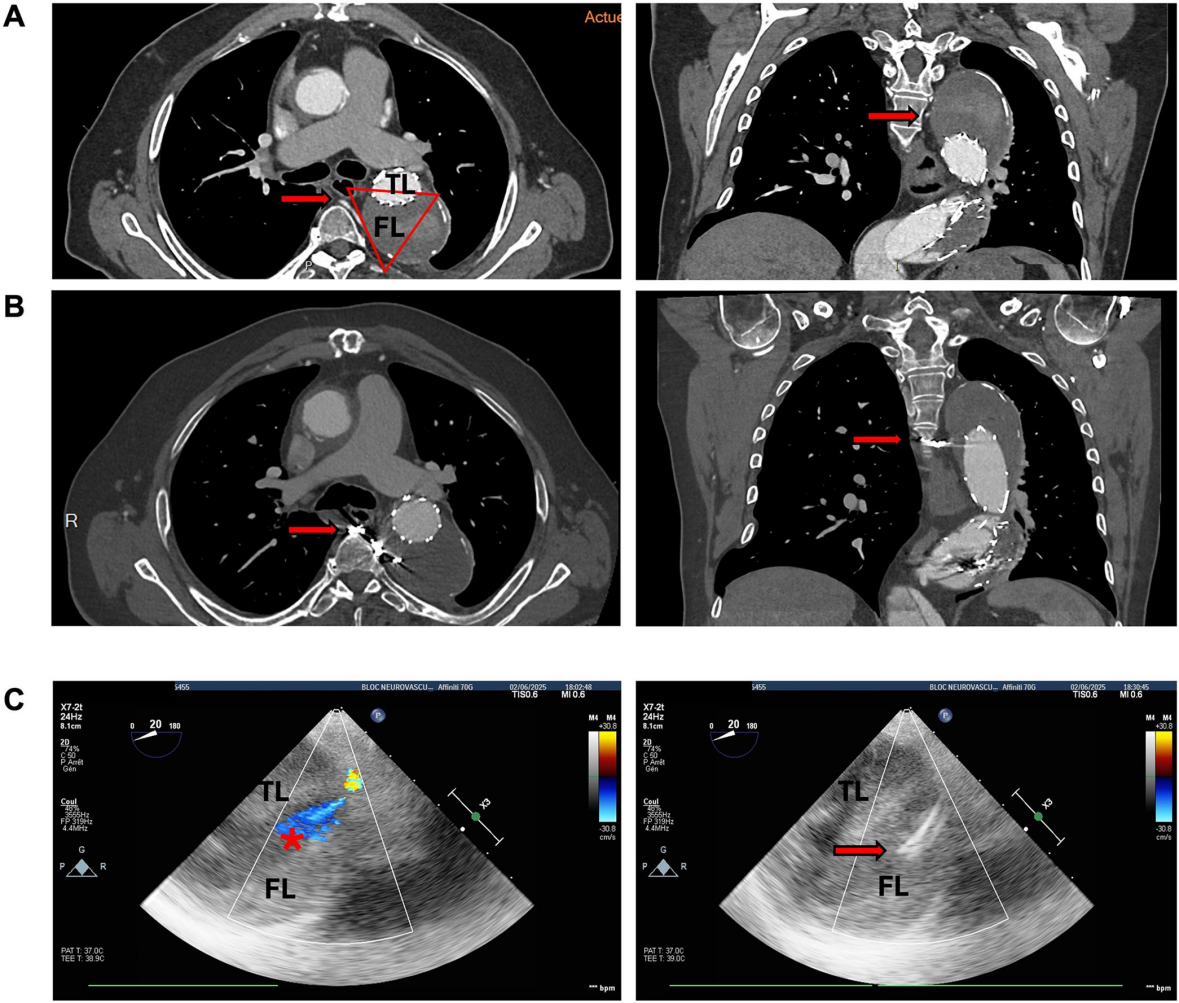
TEE-guided localisation and exclusion of an intercostal artery inflow at the T5 level: pre- and post-procedural CT and intraoperative imaging. (A) Pre-procedural contrast-enhanced computed tomography angiography (axial and coronal views) at the level of the fifth thoracic vertebra (T5), demonstrating persistent false lumen perfusion of the descending thoracic aorta related to a patent intercostal artery (red arrows). The red triangle indicates TEE probe orientation. The true lumen (TL) and false lumen (FL) are indicated. (B) Post-embolisation contrast-enhanced CT images at the same vertebral level (T5), showing microcoil material within the proximal intercostal artery (red arrows) with exclusion of false lumen perfusion. (C) Intraoperative transoesophageal echocardiography (TEE), short-axis views of the descending thoracic aorta at the corresponding T5 level. The TEE probe was positioned in the oesophagus at approximately 30 cm from the dental arch. The true lumen (TL), false lumen (FL). Top left: color Doppler demonstrates a focal inflow jet from the intercostal artery into the false lumen (red asterisk). Top right: coil artefact at the origin of the intercostal artery after selective embolisation (red arrow). Absence of residual colour Doppler signal within the false lumen, confirming complete flow cessation after embolisation.
